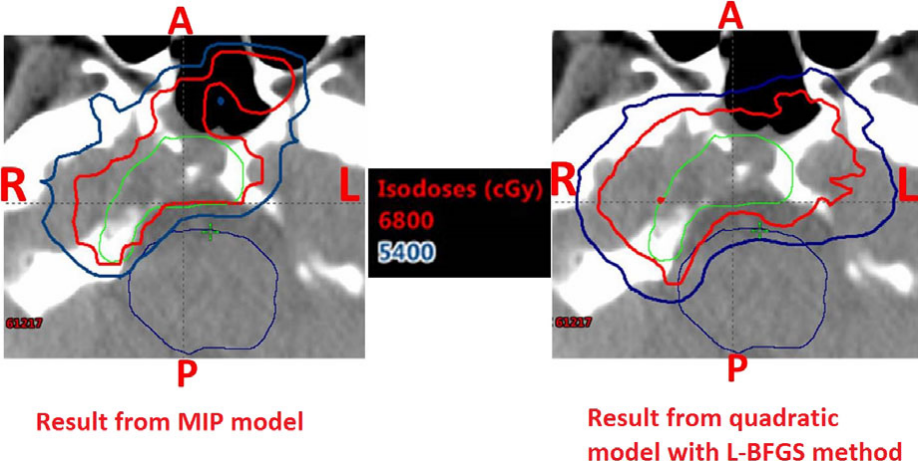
Dose distribution results at one CT slice for patient 2: MIP model with dose‐volume constraints (left) *and quadratic model solved by* L‐BFGS method (right). Green: Clinical target volume (CTV), Cyan: Brain stem. Red: prescription iso‐dose line (68 Gy[RBE]), Dark Blue: iso‐dose line of 54 Gy[RBE]. MIP model with dose‐volume constraints generated a plan with much better brain stem protection at the cost of slight tumor underdosage.
